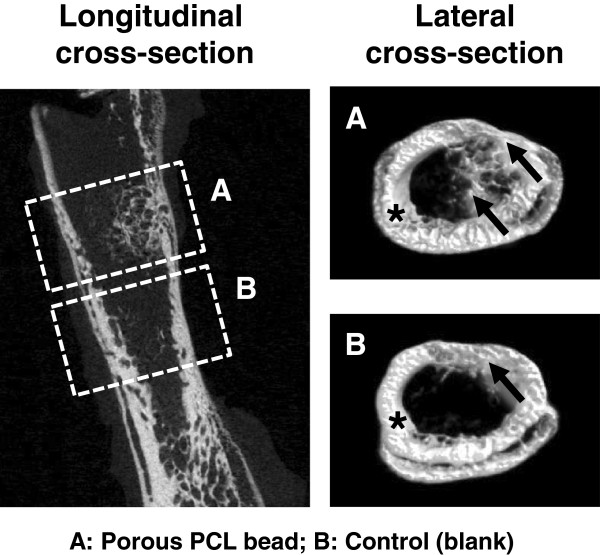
μ-CT images of femoral bone defect showing the mineralized bone regeneration behavior of (A) porous PCL beads and (B) control (blank) [*, host bone; arrow, regenerated (mineralized) bone].
